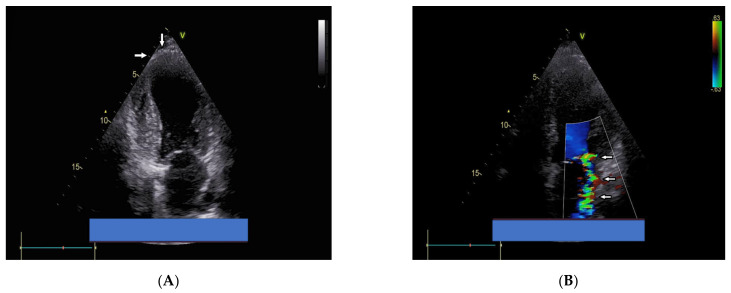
Admission echocardiography. (A): Apical left ventricle aneurysm (white arrows). (B): Secondary mitral regurgitation with eccentric jet due to the posterior cusp restriction (white arrows).
